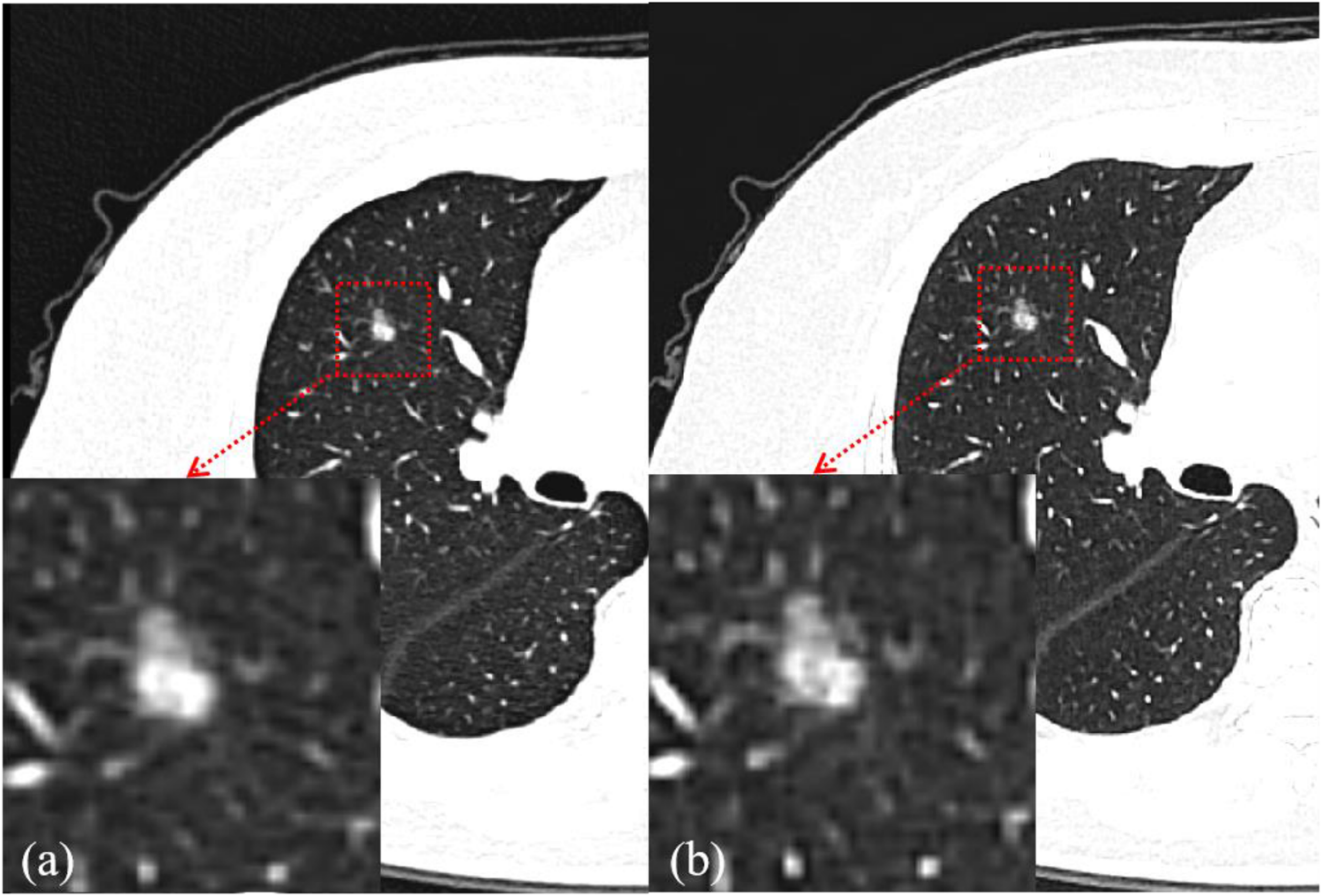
Hybrid iterative reconstruction (HIR) (a) and artificial intelligence iterative reconstruction (AIIR) (b) in routine‐dose Computed tomography (CT) performed on a 69‐year‐old woman with malignant pulmonary nodule (adenocarcinoma) in right lobe. Compared with the HIR algorithm, the contours of the nodule is clearer, the lung texture is more distinct, and the noise is less in images reconstructed by AIIR algorithm.
